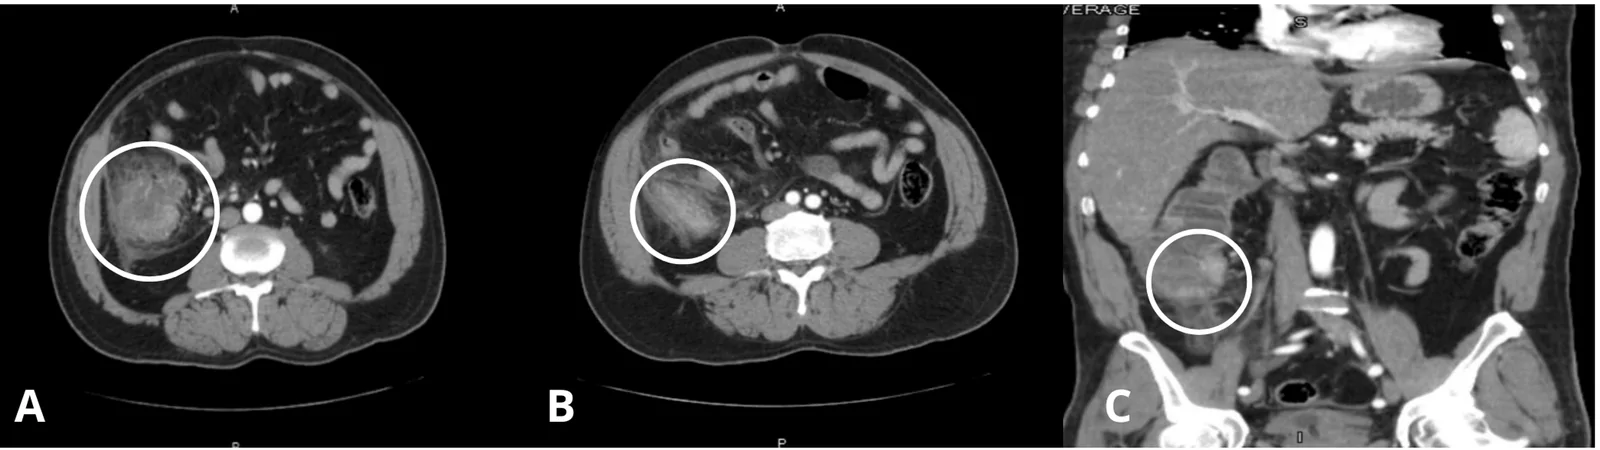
Contrast-enhanced abdominal CT showing the appendiceal mass at presentation CT: computed tomography CT demonstrating a heterogeneous 7 cm mass (white circle) in the right hemiabdomen with central hypoattenuation, irregular peripheral enhancement, and marked surrounding inflammatory fat stranding. (A, B) Axial views at the level of the cecum show the mass abutting the ascending colon/hepatic flexure and the second portion of the duodenum, although its exact organ of origin could not be definitively established on imaging. (C) Coronal reformat confirms the craniocaudal extent of the mass and its relationship to adjacent bowel loops. No pneumoperitoneum or significant free intraperitoneal fluid is evident
